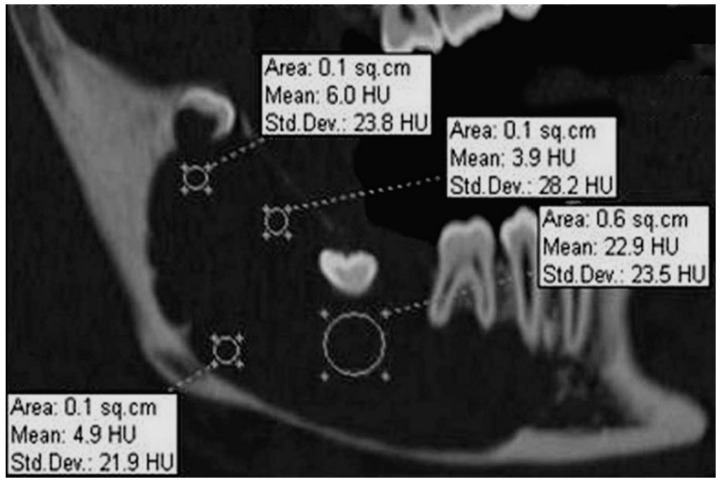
Sagital multi-slice computed tomography (MSCT) image: A large uniloculated lesion involving impacted mandibular right second molar. The lesion attenuation coefficient varies from 3.9 to 22.9 HU.
